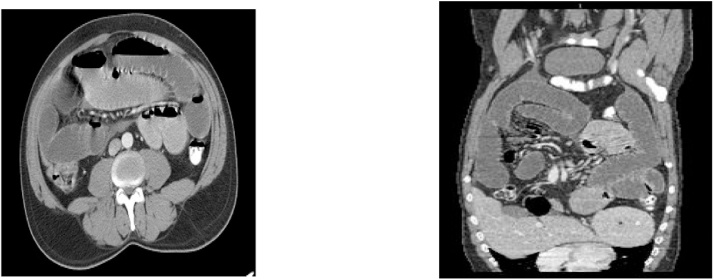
CT abdomen showing Small bowel complete obstruction with transitional zone seen distal ilealloops.
